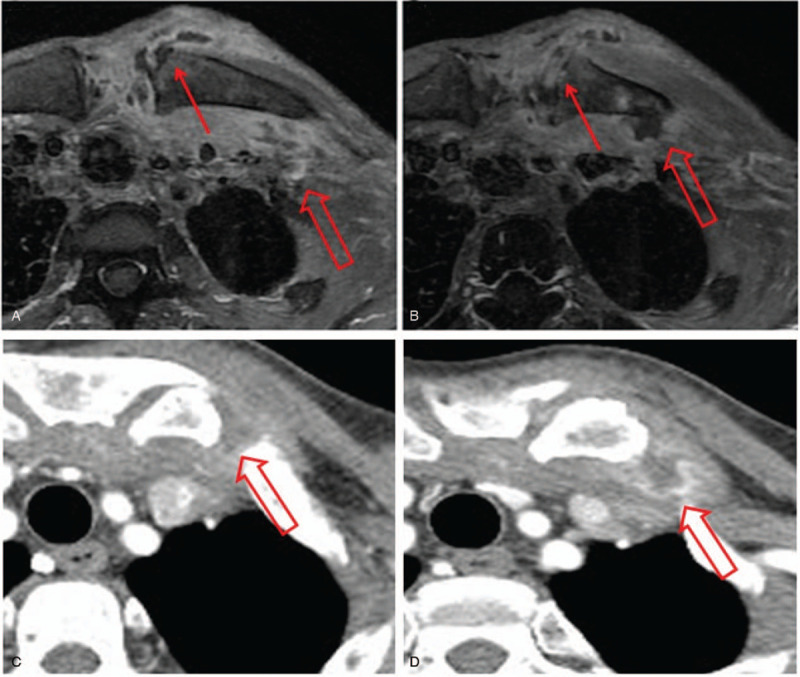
(A and B) Fat suppression phase of magnetic resonance imaging demonstrates left SCJ infectious arthritis with multiple small abscesses at the subcutaneous layer (arrow), and abscess pockets posterior to the clavicle (empty arrow). (C and D) Contrast enhanced chest computed tomography was performed after four weeks of antibiotic treatment. Decreased size of abscess pockets posterior to the clavicle (empty arrow) is observed.
